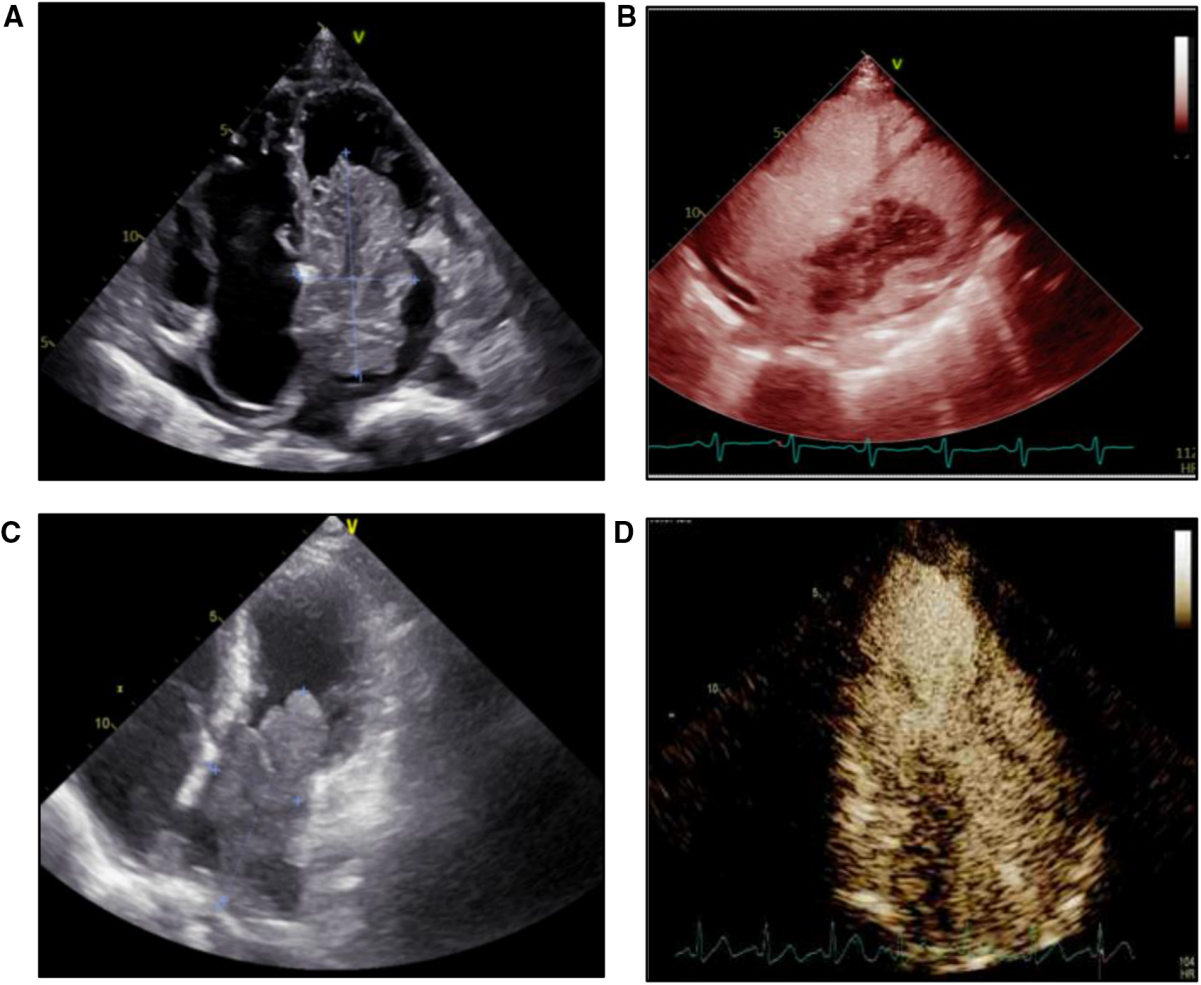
Echocardiography and microbubble contrast-enhanced echocardiography images of patients with benign or malignant primary atrial tumor. (A) Echocardiography image of a patient with cardiac myxoma. (B) Microbubble contrast-enhanced echocardiography image of a patient with cardiac myxoma. (C) Echocardiography image of a patient with intimal sarcoma. (D) Microbubble contrast-enhanced echocardiography image of a patient with intimal sarcoma.
